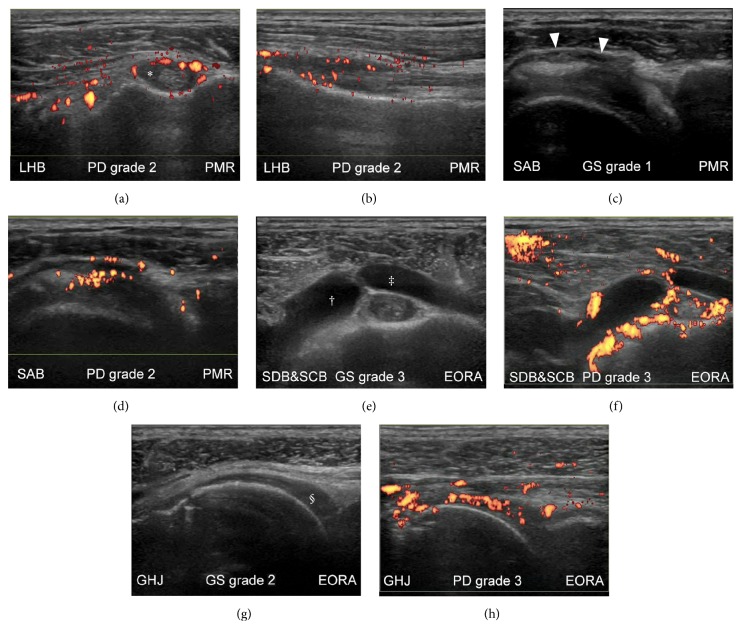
Representative ultrasound (US) images for grading of synovial pathology of the shoulder. Images from the shoulders of patients with polymyalgia rheumatica (PMR) (a–d) and elderly-onset rheumatoid arthritis (EORA) with PMR-like onset (e–h) are shown on gray-scale (c, e, and g) and power Doppler (a, b, d, f, and h) ultrasonograms. The shoulder was in a neutral position (a, b, e, f, and h), in a modified Crass position (c, d), or internally rotated (g). Moderate hyperemia was detected in the long head of biceps tendon sheath (*∗*) on transverse (a) and longitudinal (b) scans. Mild synovial effusion (c) and moderate hyperemia (d) were detected in the subacromial bursa (arrowheads). Severe synovial effusion (e) and severe hyperemia (f) were detected in the subcoracoid bursa (†) and subdeltoid bursa (‡). Moderate synovial proliferation (g) and severe hyperemia (h) were detected in the glenohumeral joint (§).
